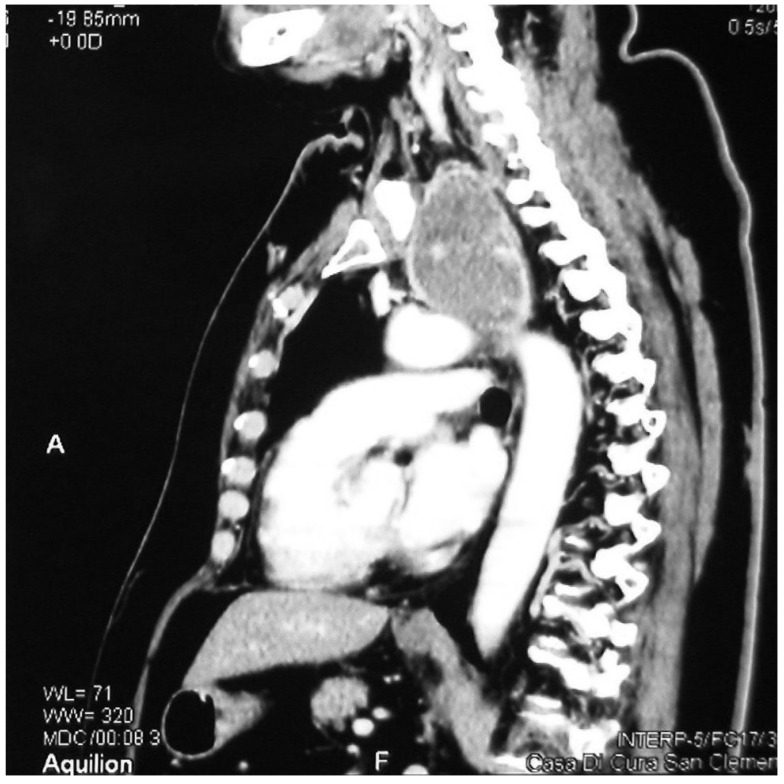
Sagittal CT showing a retrovascular retrosternal goitre.
